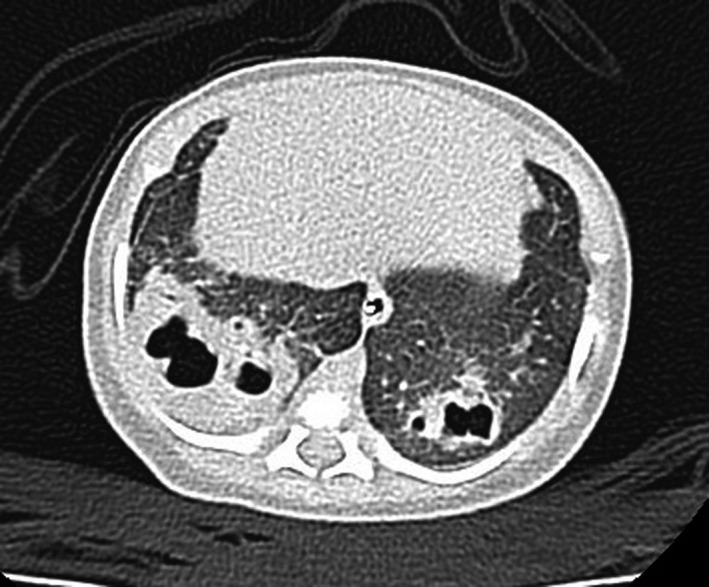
Pulmonary CT scan. Multiple thick‐walled cystic images within bilateral basal condensation and in the right upper lobe and pneumatoceles in the context of necrotizing pneumonia
